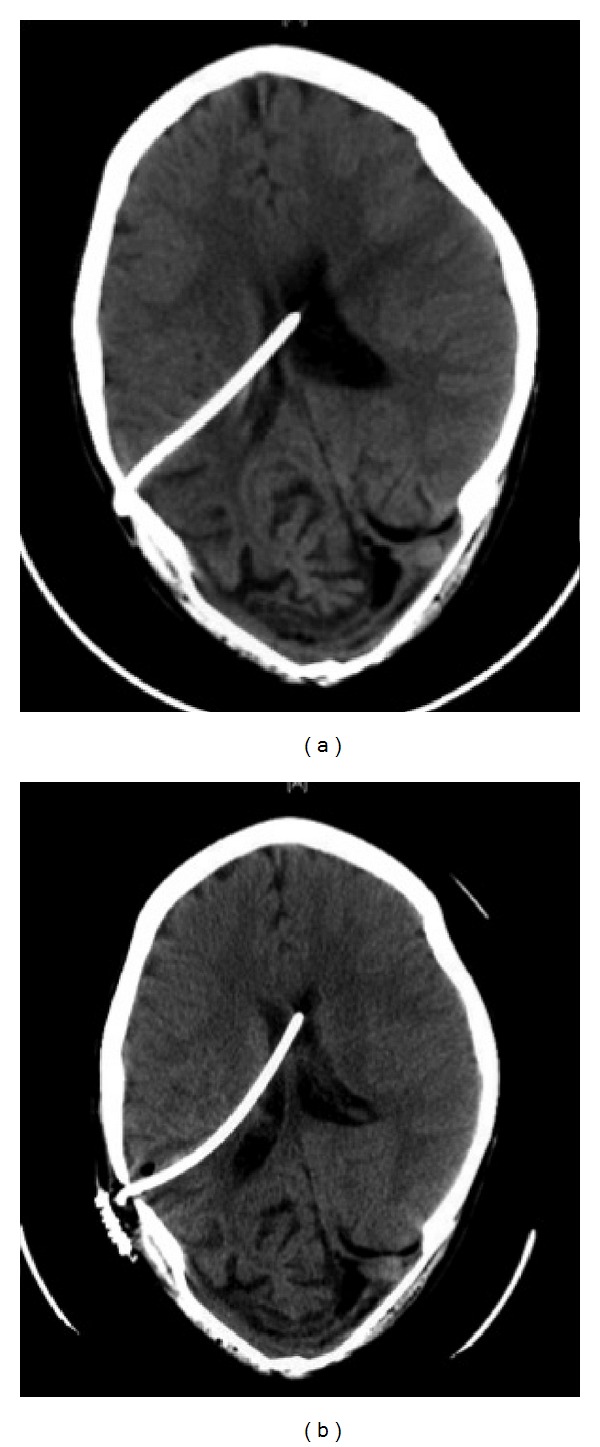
Computed Tomography of the brain demonstrating dilated ventricular system as a sign of ventriculoperitoneal shunt malfunction (a) and the reduction in ventricular size after VP shunt revision (b).
